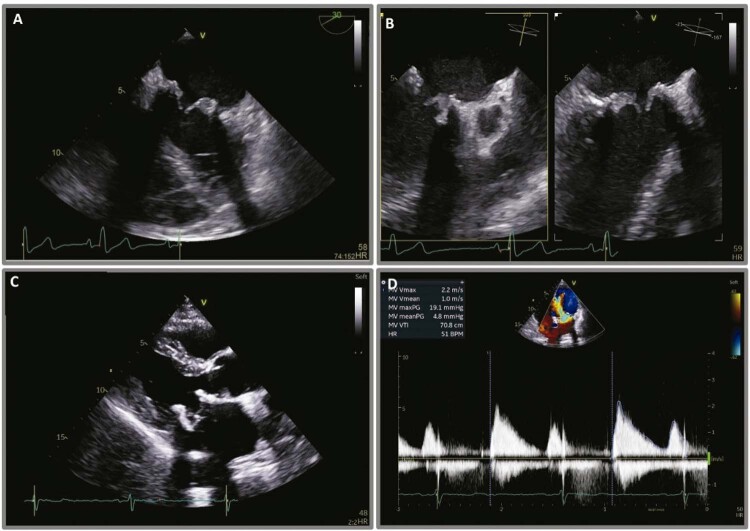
(A) e (B) - Vistas diferentes de ecocardiografia transesofágica realizada no 10º dia de anticoagulação mostrando folhetos mais finos. (C) Ecocardiografia transtorácica realizada 15 dias após a alta, mostrando espessura normal da prótese valvar biológica. (D) Doppler de onda contínua documentando um gradiente médio de pressão transvalvar de 5 mmHg.
